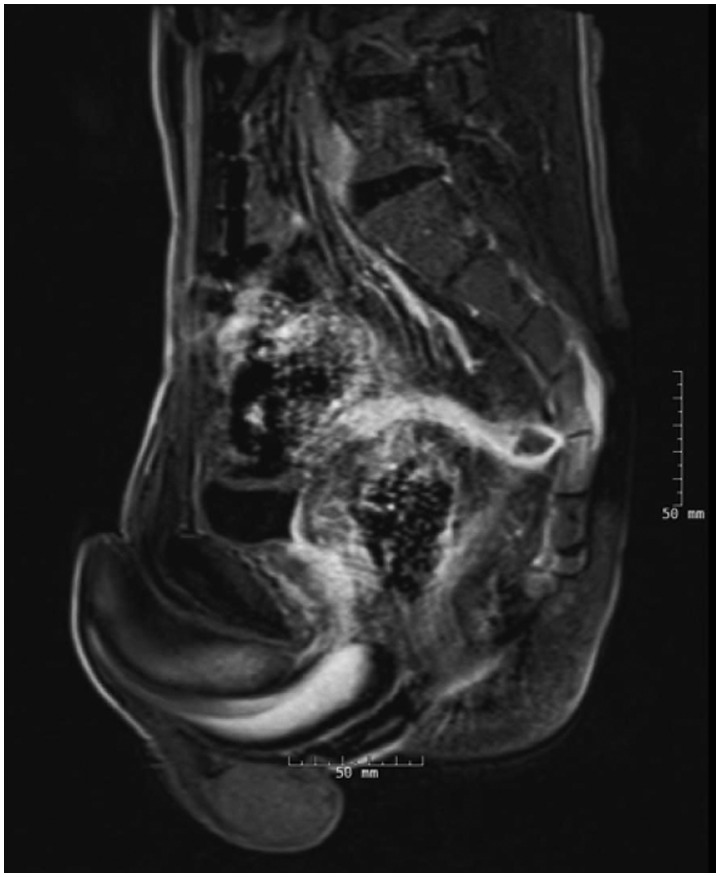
MRI images showing a fluid-containing tract extending from the presacral region at approximately the S2–S3 level. The tract extends anteriorly and encircles the mid portion of the pouch, well above the level of the anastomosis. No definite connection into the pouch is seen. The collection terminating in the presacral space measured 2.0 × 1.1 × 1.6 cm, with peripheral enhancement from active inflammation.
